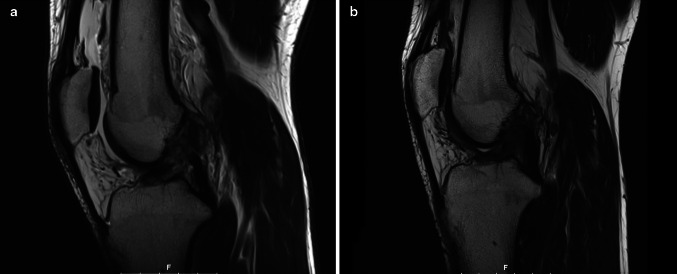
Lateral MRI showing the torn ACL immediately after injury and at 12 months follow-up (after removal of screw)
